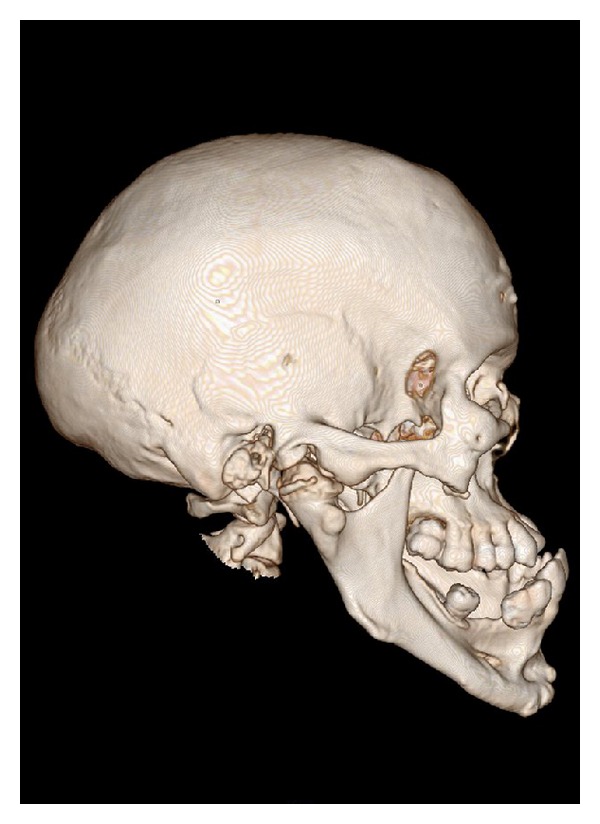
Lateral view of 3D volume rendered CT scan showing enlarged right condyle, coronoid process, mandibular body, and zygoma.
